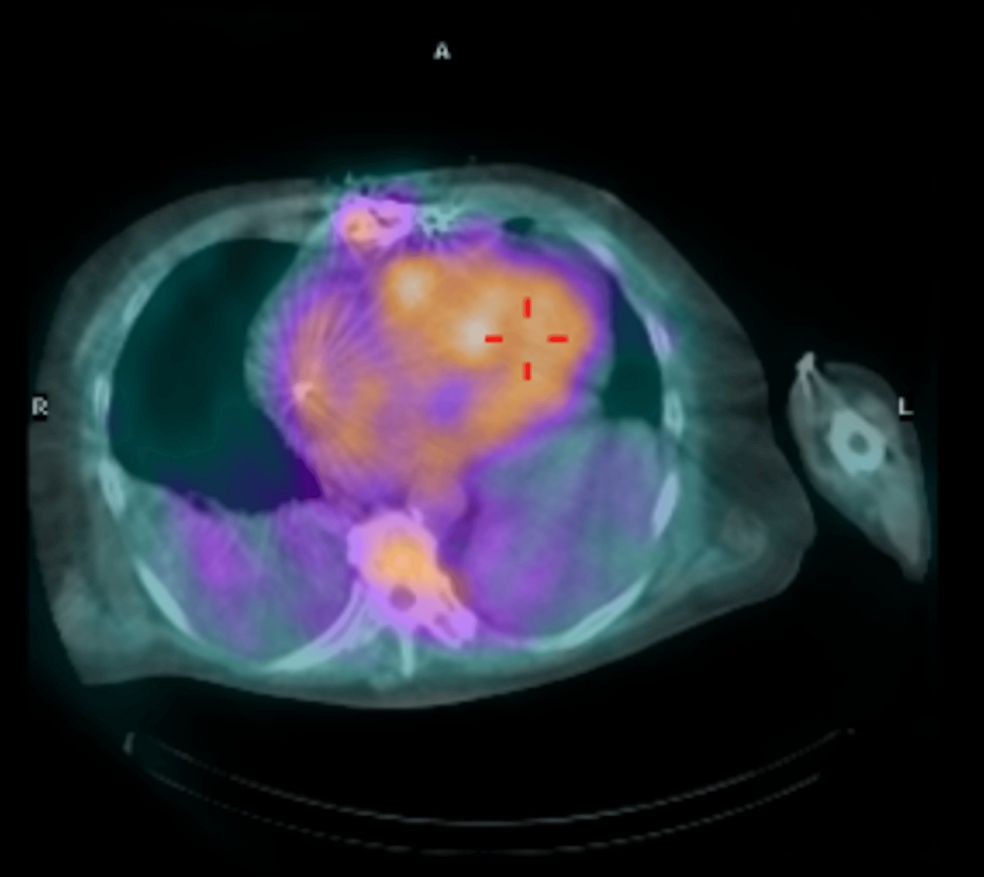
Axial projection of fused SPECT/CT images displaying intense Tc99PYP tracer uptake in the interventricular septum, mild uptake in the left ventricle lateral wall, and mild uptake in the right ventricular free wall SPECT/CT images acquired 60 minutes after the injection of radiotracer. SPECT: Single-photon emission computed tomography; Tc99m PYP: technetium pyrophosphate
